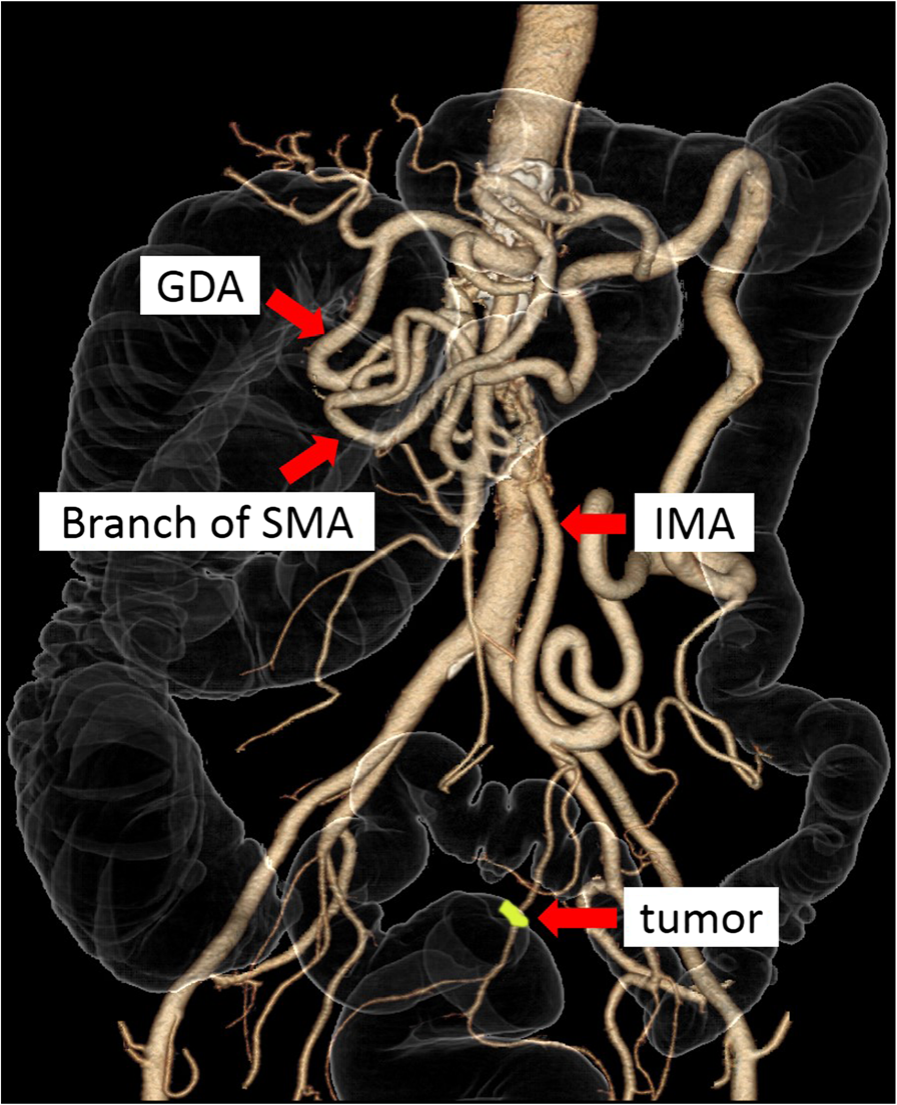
Merged image of computed tomography angiography and colonography. Computed tomography angiography revealed abdominal aortic locoregional stenosis. Accompanying the descending colon, collateral circulations comprised the gastroduodenal artery (GDA) and superior mesenteric artery (SMA), with flow into the inferior mesenteric artery (IMA), and deficiency of the left colic artery
